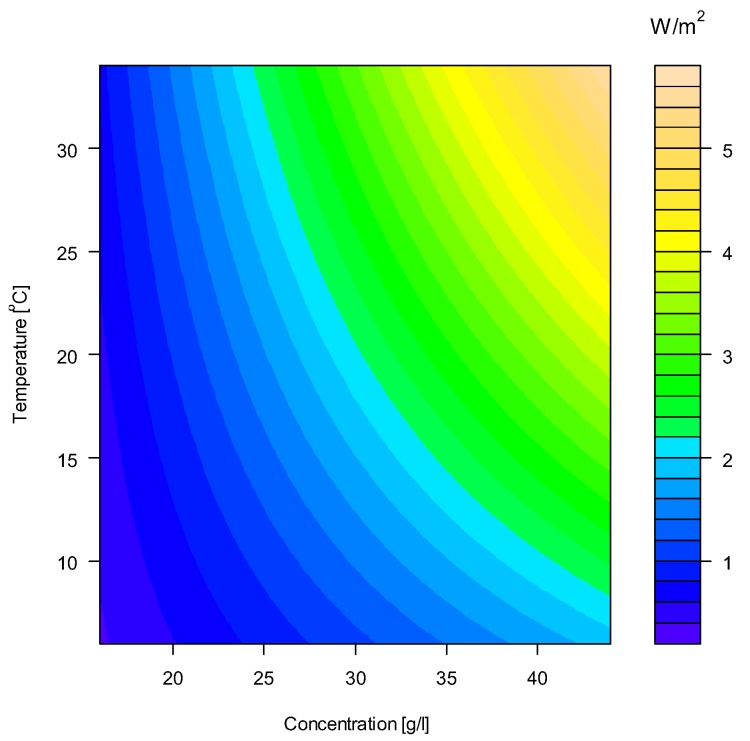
Contour plot of the specific power (W/m^2^) as function of concentration and temperature for the CTA membrane. Note that the operating pressure has been optimized for each combination of concentration and temperature.
